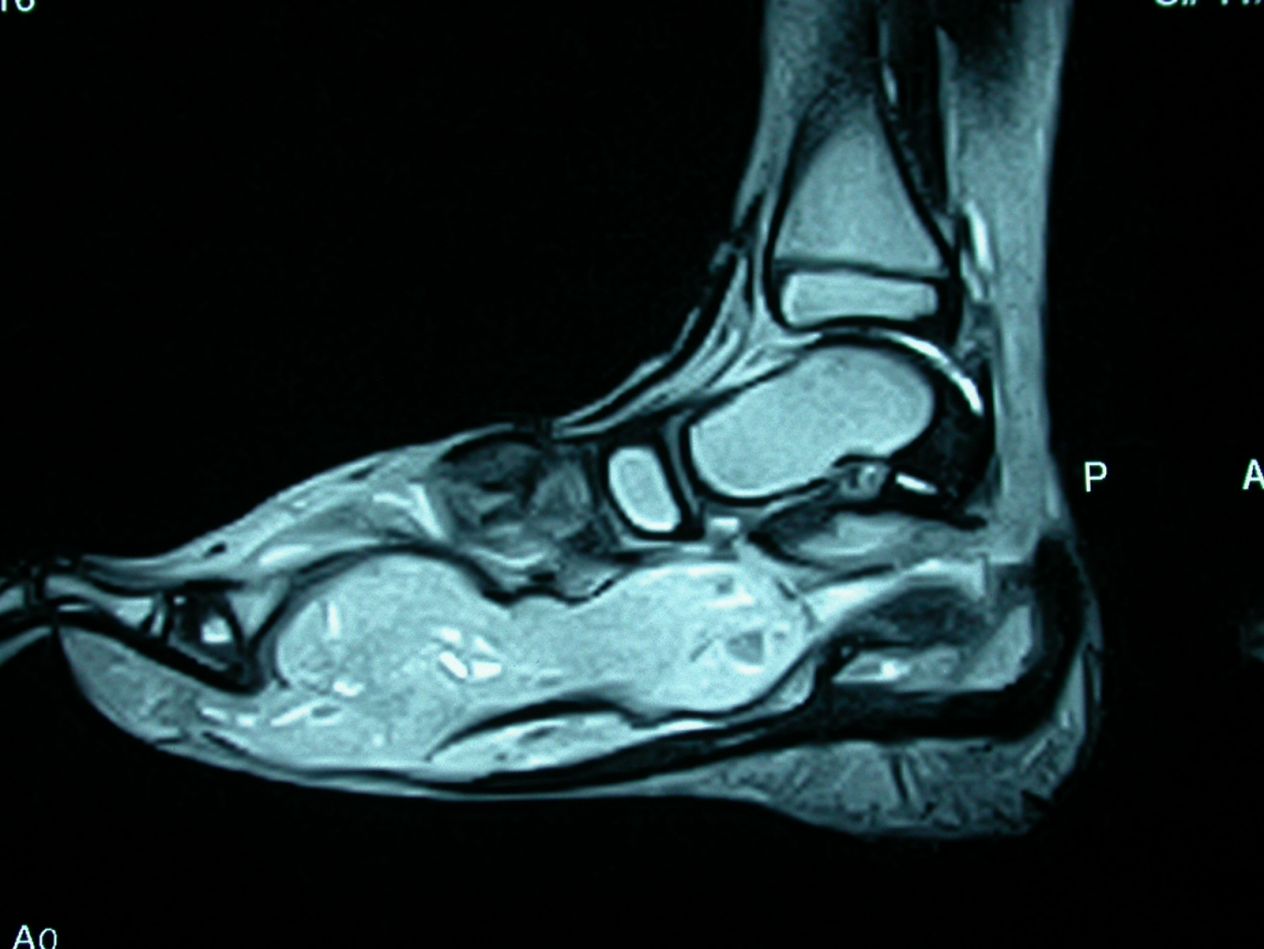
MRI showing large cold abscess on the planter surface.
